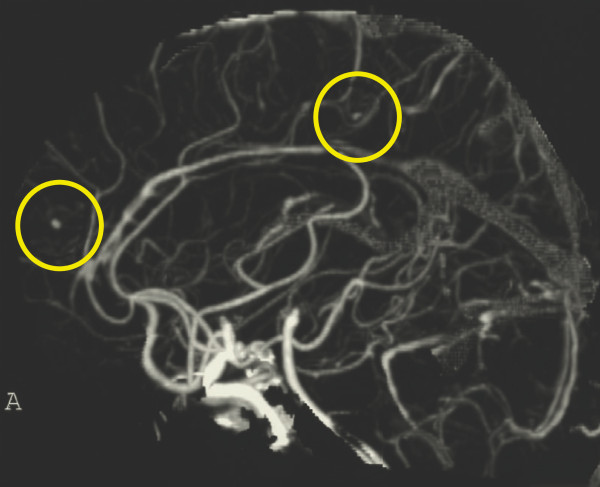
Three-dimensional computed tomography angiography. The two yellow circles indicate cerebral mycotic aneurysms.
